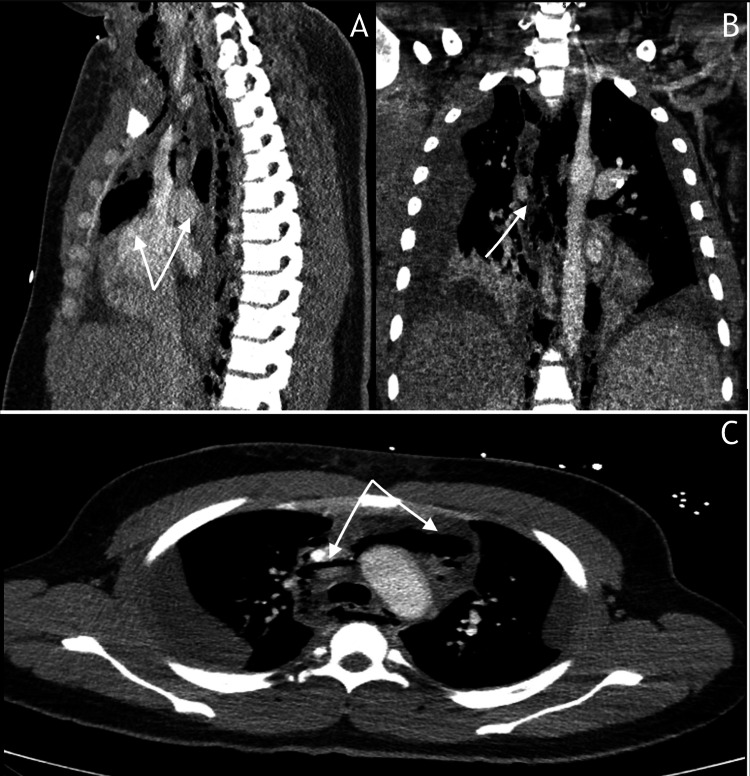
Initial computed tomography (CT) chest Imaging demonstrates pneumomediastinum and subcutaneous emphysema. (A) Sagittal view showing air tracking along fascial planes from the submandibular space to the superior mediastinum. (B) Coronal reconstruction reveals bilateral cervical subcutaneous emphysema extending to the thoracic inlet. (C) Axial section at the T3 level demonstrating mediastinal air surrounding the great vessels and trachea, with associated small bilateral pleural effusions
